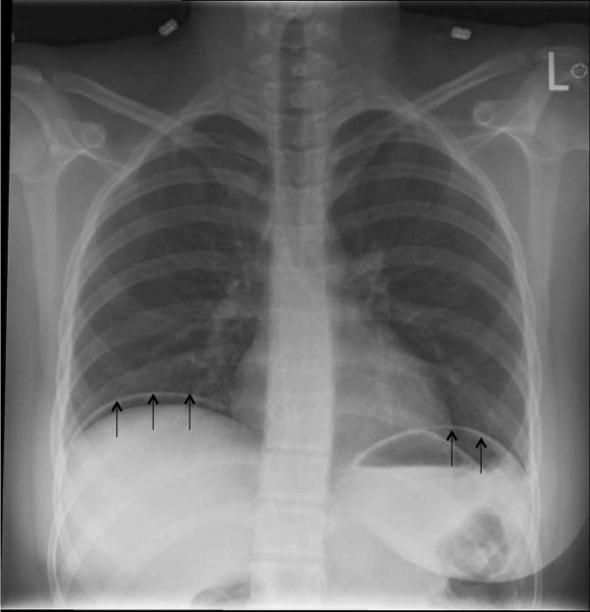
Chest radiograph demonstrating free intra-abdominal air below the diaphragm. (Black arrows demonstrate the air below the diaphragm).
